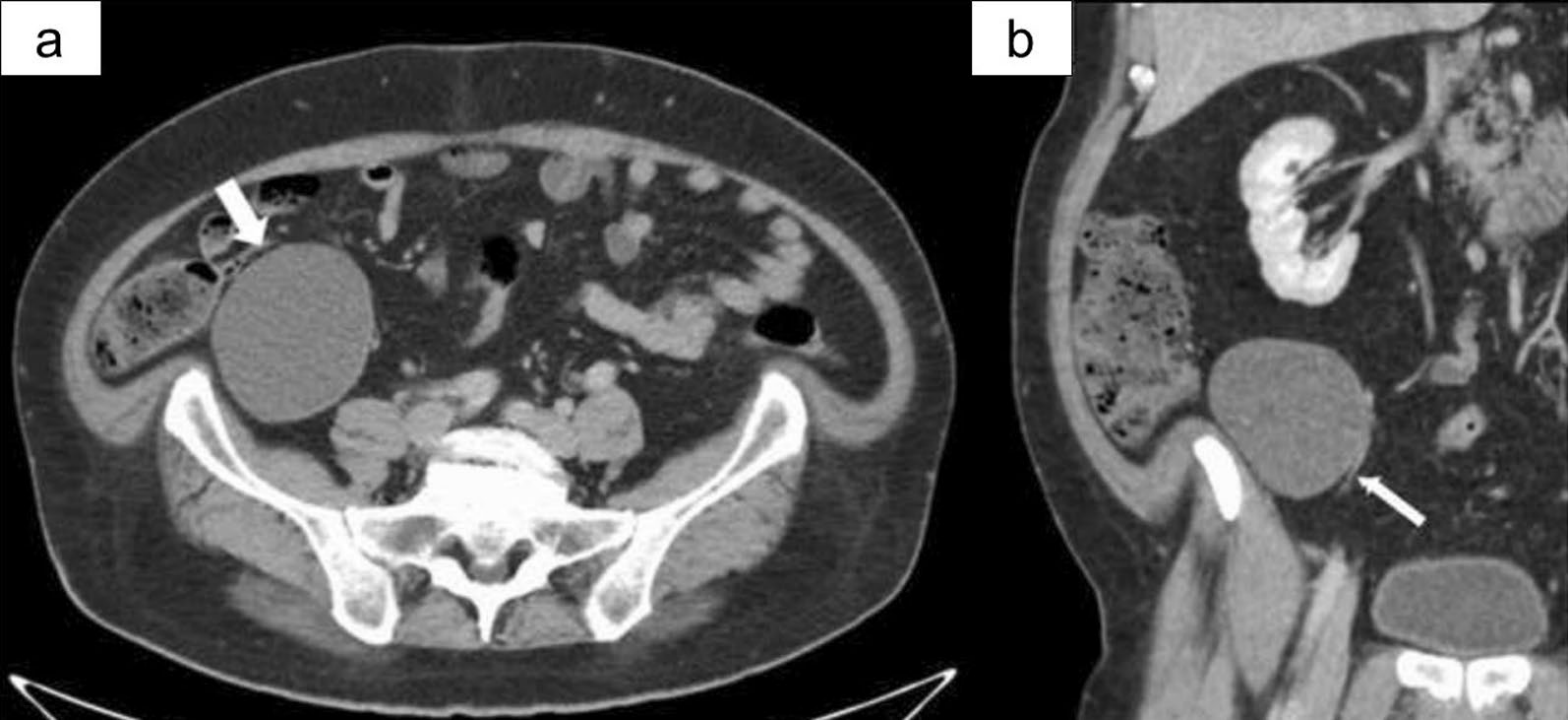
Preoperative computed tomography. Enhanced computed tomography showed a unilocular cystic mass (thick arrow) of 10 cm in diameter located below the right kidney in the retroperitoneum (a), which appeared to be close to the appendix on the ventral side and to the right gonadal vessel (thin arrow) on the medial side (b)
